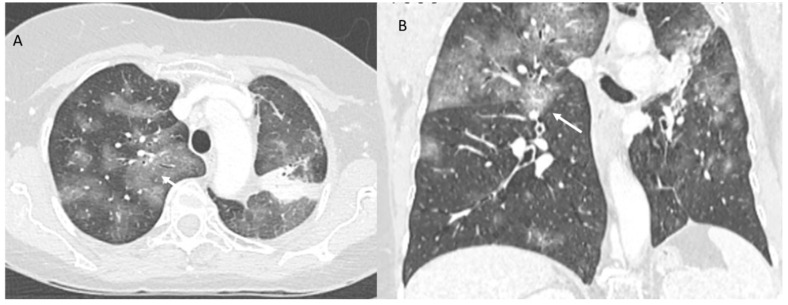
ICI-related pneumonitis. AIP-ARDS pattern on CT (axial: (A) and coronal: (B)): diffuse ground-glass opacities involving a majority of the lungs (arrows), although areas of lobular sparing can be detected.
